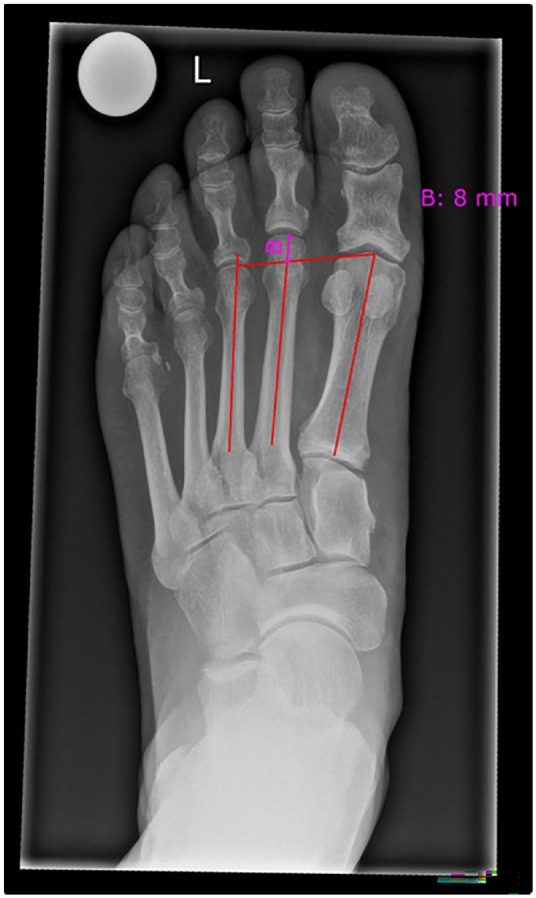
A radiograph of the left foot (dorsoplantar view, weightbearing) of a patient who is to undergo first-ray amputation. Visualization of the Coughlin method marked with red lines and measured in bright pink (“B”). [See online article for color figure.]
